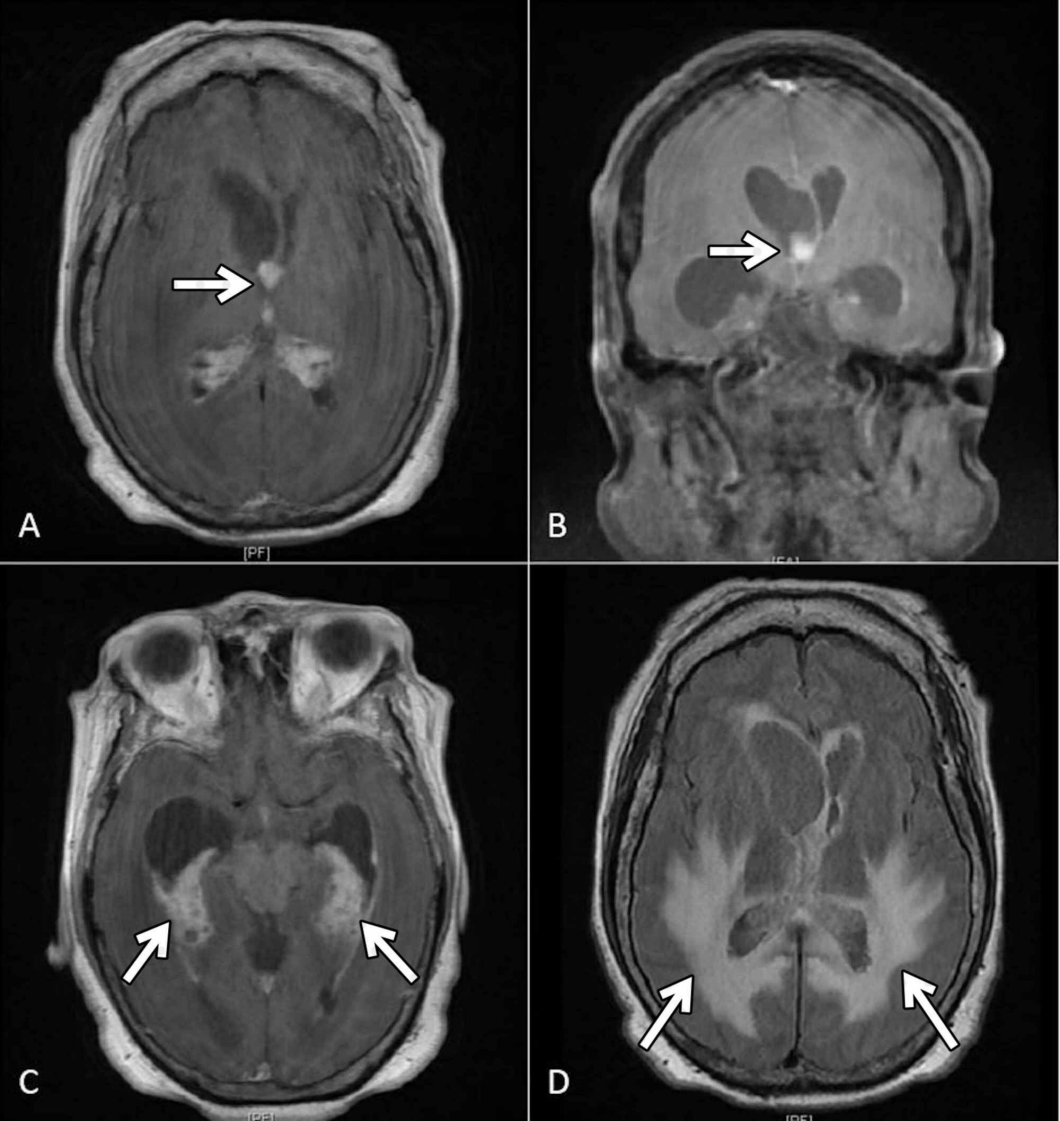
MRI Findings of Choroid Plexitis (A) Axial T1-weighted post contrast MRI and (B) coronal T1-weighted post contrast MRI demonstrating a hyperintense nodularity at the foramen of Monro (arrows). (C) Axial T1-weighted post contrast MRI demonstrating involvement of the choroid plexus within the temporal horn of the lateral ventricle (arrows). (D) Axial section of T2-weighted FLAIR MRI demonstrating transependymal flow (arrows). FLAIR, Fluid-attenuated inversion recovery.
